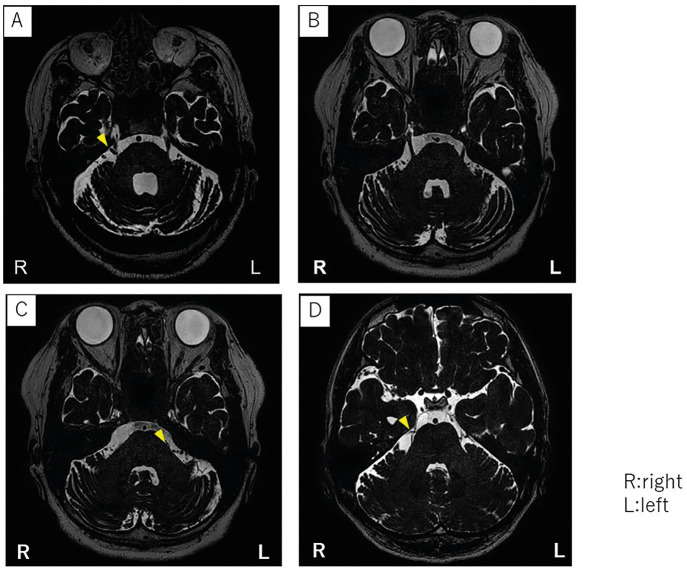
A-D) MRI scans showing compression of the trigeminal nerve by the superior cerebellar artery (SCA). R: right, L: left.
